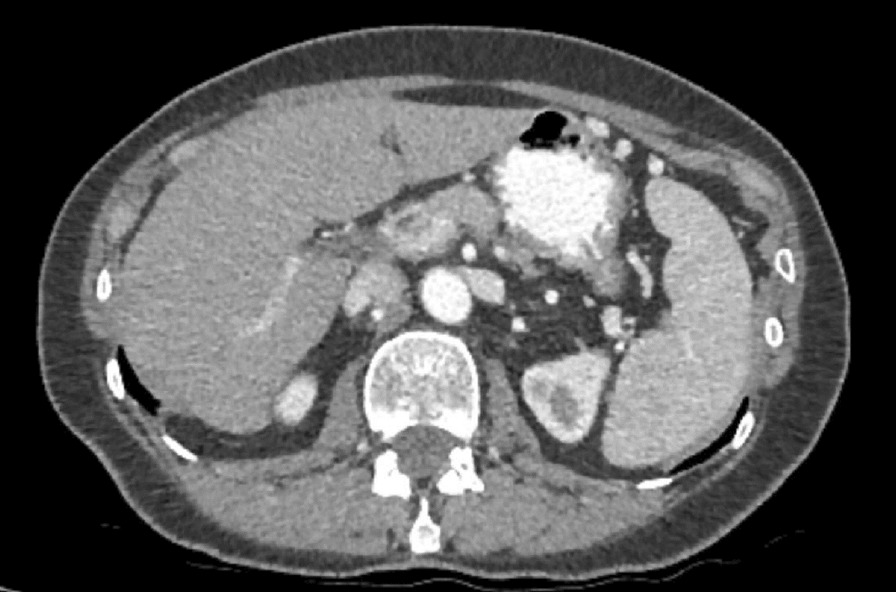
Posttreatment CT showing no residual pancreatic tumor
